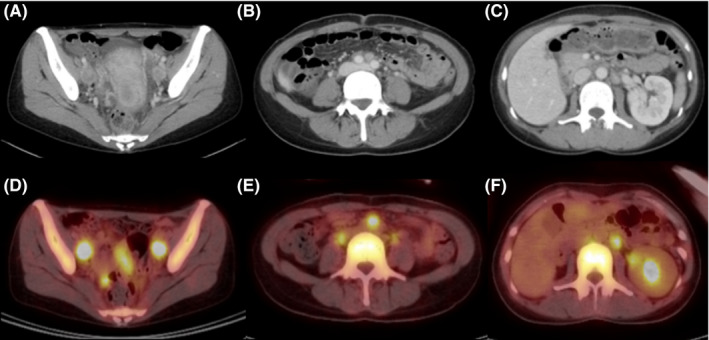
Computed tomography (CT) and ^18^fluoro‐2‐deoxyglucose‐positron emission tomography (FDG‐PET) showing multiple lymphadenopathy before the initiation of chemoradiotherapy. A‐C, CT image showing enlarged lymph nodes in the external iliac, common iliac, and para‐aortic lymph node regions, respectively. D‐F, FDG‐PET images corresponding to A‐C
